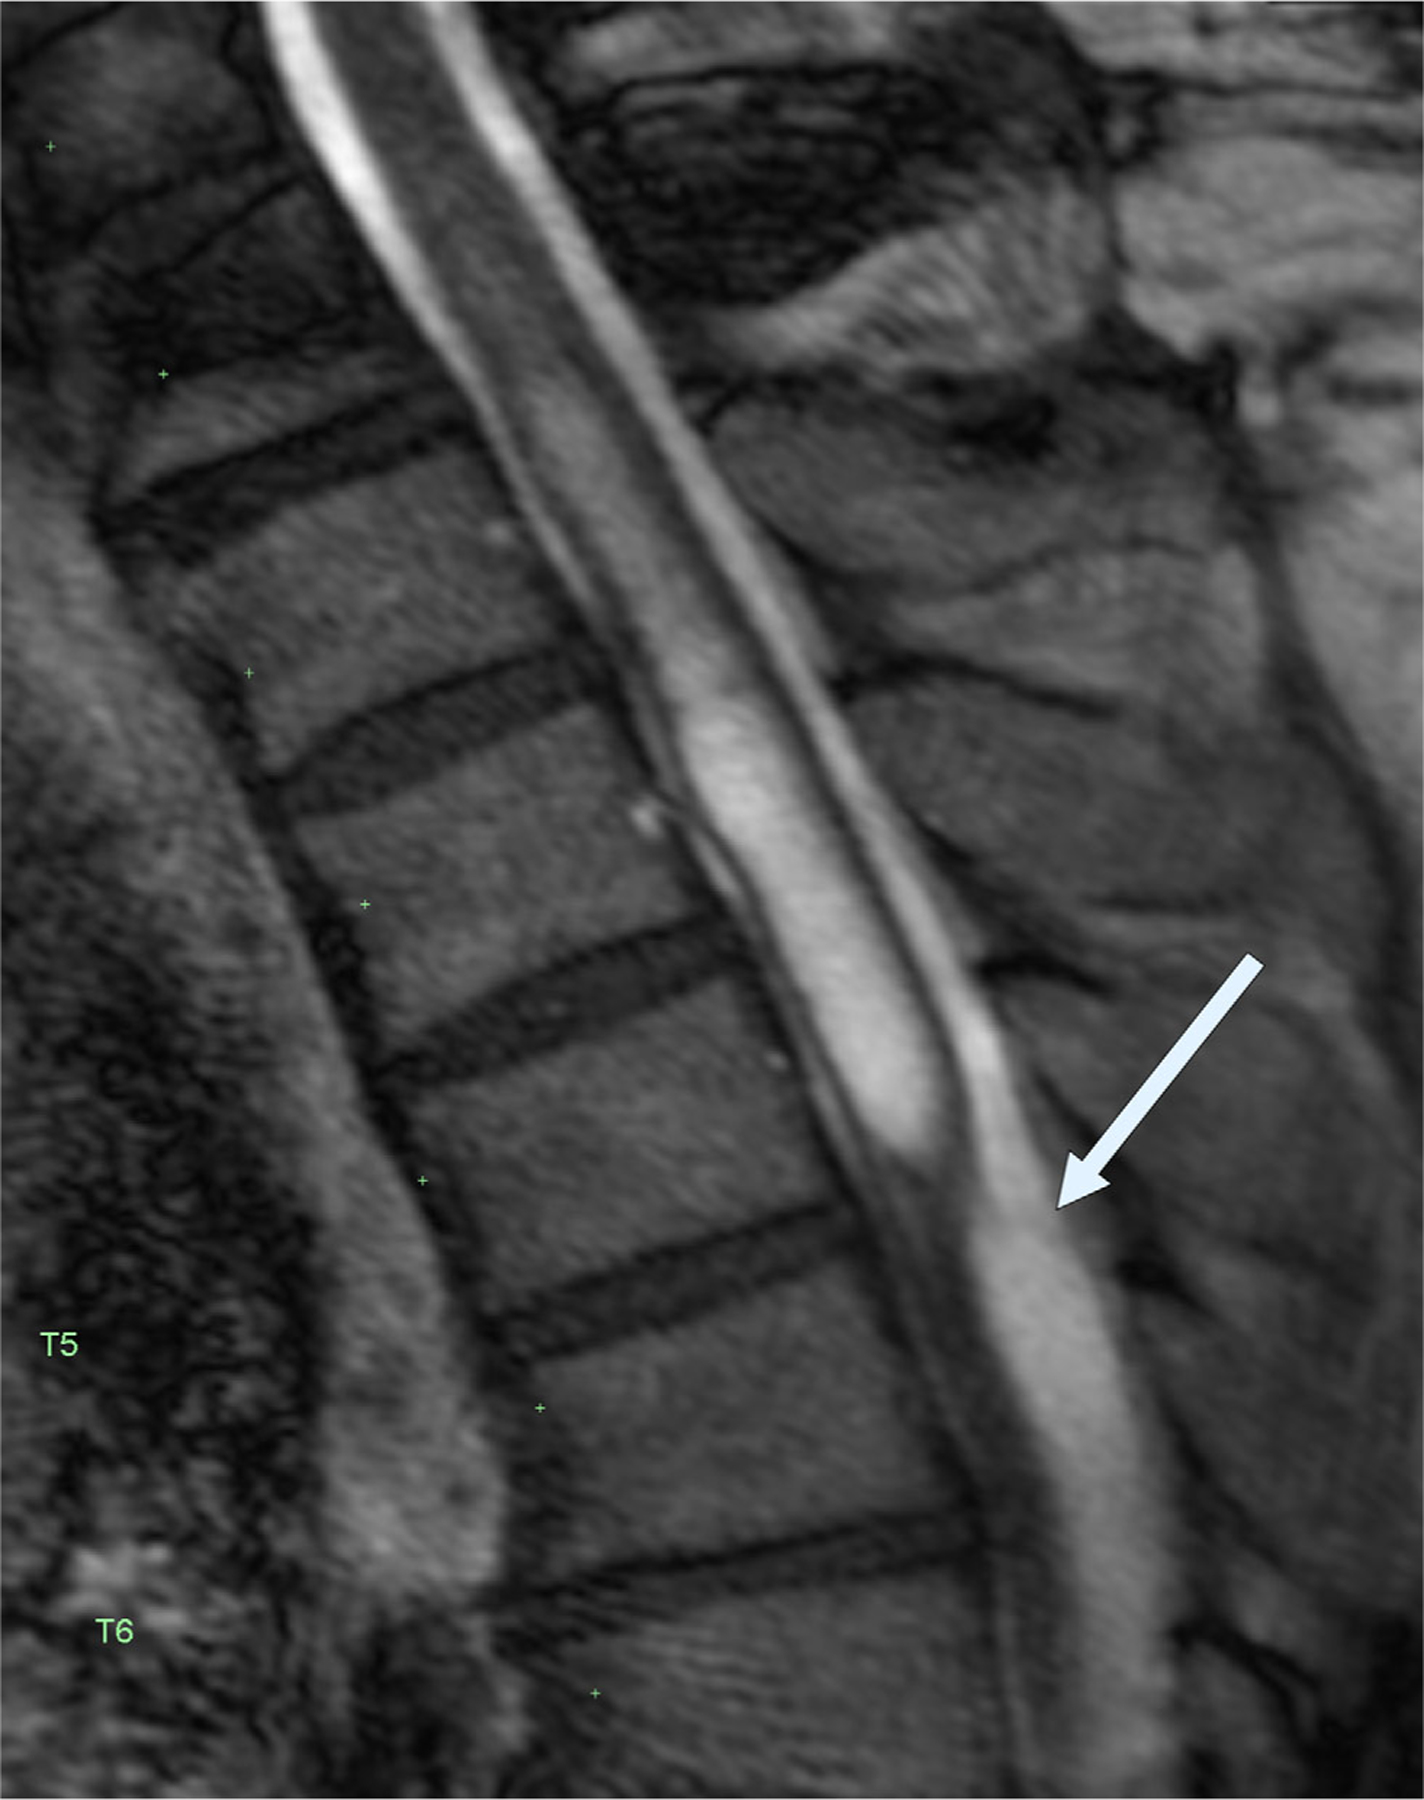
Still image from cardiac-gated, heavily T2-weighted sagittal spine MRI demonstrating an arachnoid membrane (arrow) that was the cause of a spinal cord syrinx. The membrane was not detectable on standard T2 MRI.
